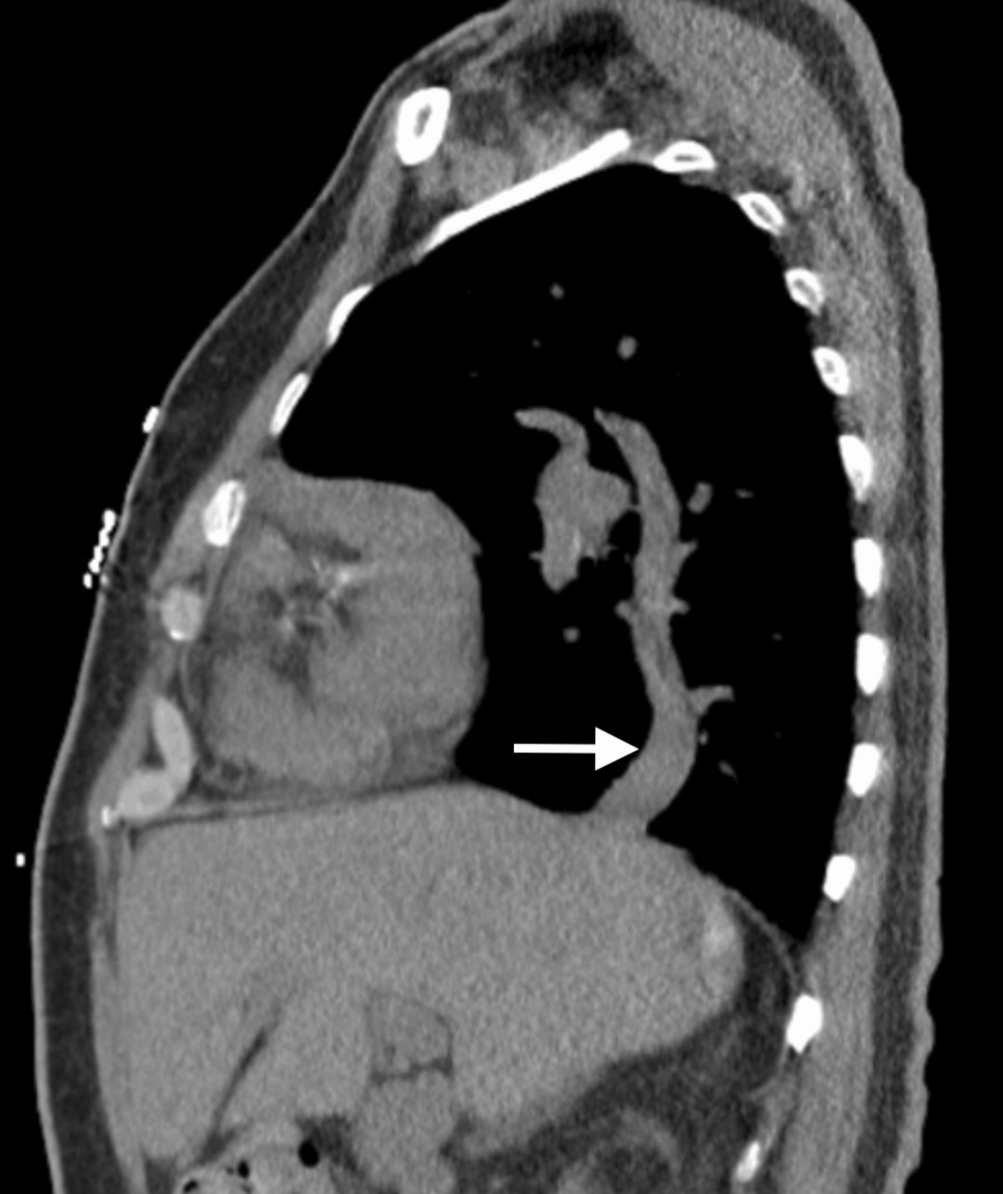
Chest computed tomography scan without contrast (sagittal reconstruction) demonstrating the scimitar vein, the aberrant connection of the pulmonary vein to the IVC
